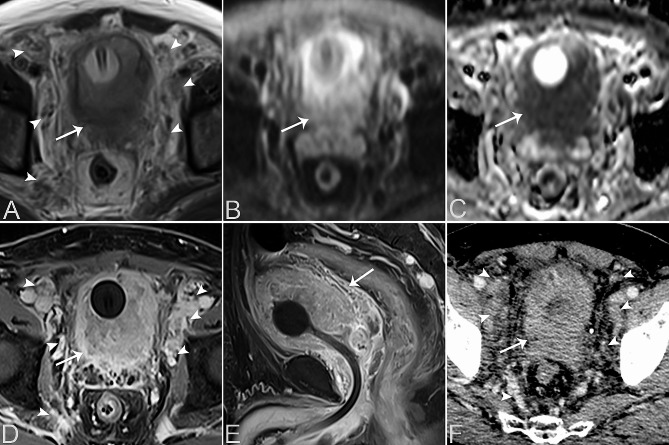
A 63-year-old male patient with bladder SUC involving the entire bladder wall diffusely. Axial T2WI (A) shows an ill-defined, irregular mass in the diffuse bladder wall, with the tumor demonstrating iso- or slight hyperintensity on T2WI (arrow). The structure of the bladder wall was blurred with unclear edges. Multiple cord-like and nodular T2 hyperintense lesions were observed around the bilateral pelvic walls and iliac vessels (arrowheads). Axial DWI (B) shows the mass with high signal intensity (arrow), and the axial ADC map (C) exhibits significant diffusion restriction (arrow). Contrast-enhanced axial (D) and sagittal (E) T1WI demonstrate obvious heterogeneous and marked enhancement of the mass (arrows), with unclear borders. Axial contrast-enhanced CT image reveals a prominently enhanced tumor in (F) the delayed phase. Multiple lymph node metastases were observed around the bilateral pelvic walls and iliac vessels (arrowheads)
